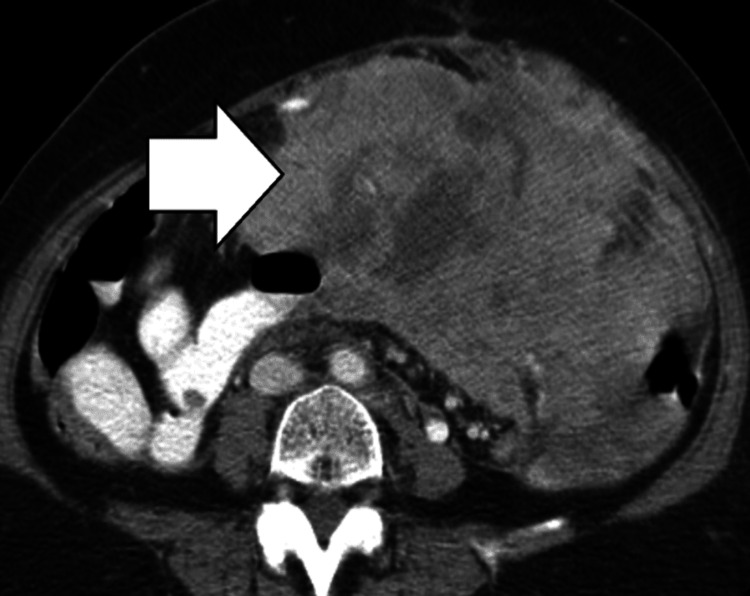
Peritoneal sarcoma. CT image shows a large heterogeneously enhancing intraperitoneal mass arising from the peritoneum (white arrow).
